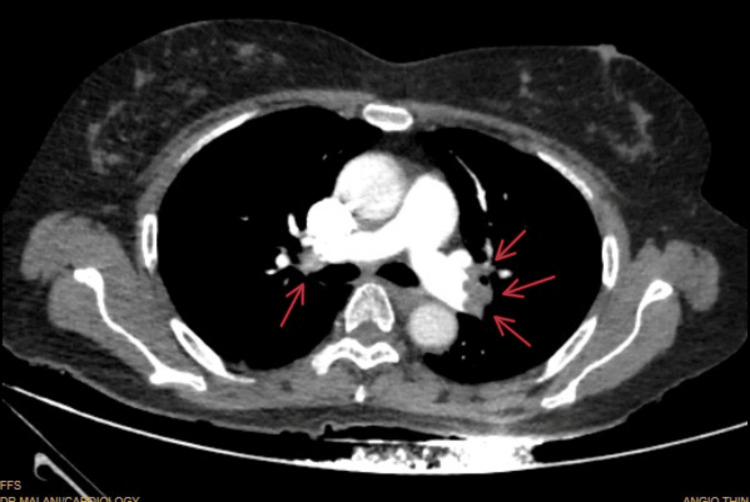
Computed tomography pulmonary angiography suggestive of bilateral pulmonary embolism.
